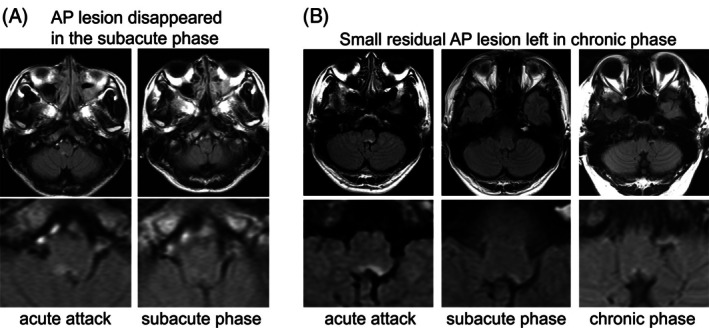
Temporal changes in MRI findings of area postrema lesions in patients with NMOSD. (A) A FLAIR hyperintensity lesion in the area postrema during an acute attack (left), disappeared after treatment (subacute phase, right). (B) A FLAIR hyperintensity lesion in the area postrema decreasing in size over time. It is still visible in the chronic phase of the disease, but is not accompanied by tissue atrophy.
